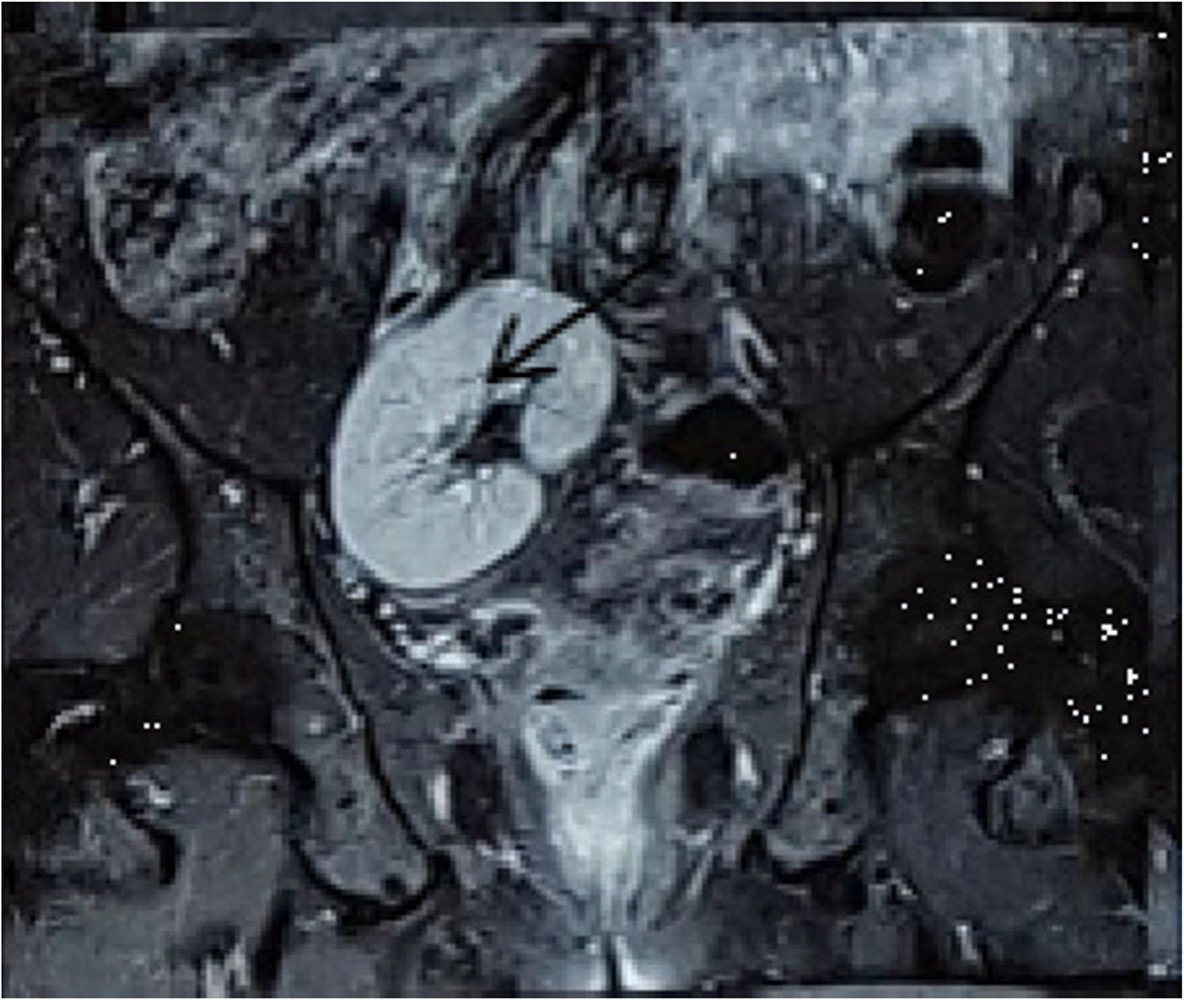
Pelvic-abdominal MRI showing the right kidney in the iliac fossa. Coronal view (Black arrow is right pelvic kidney)
